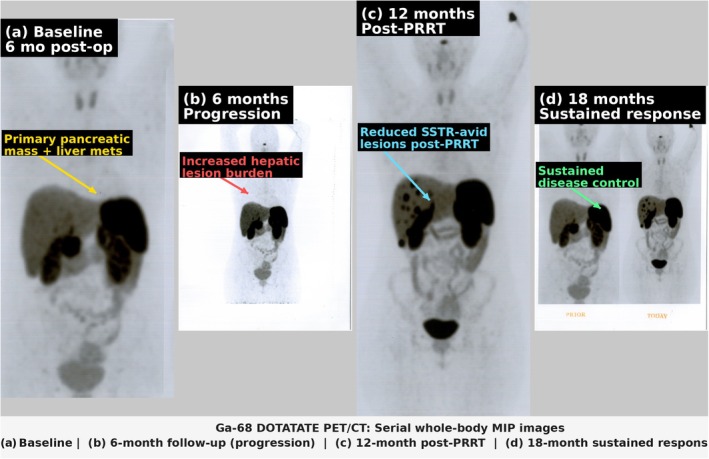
Serial follow‐up Ga‐68 DOTATATE PET CT scans showing the liver lesions and their response to treatment; (a) At the time of diagnosis the pancreatic mass, (b) 6 months later, (c) 12 months later, d: 18 months later.
